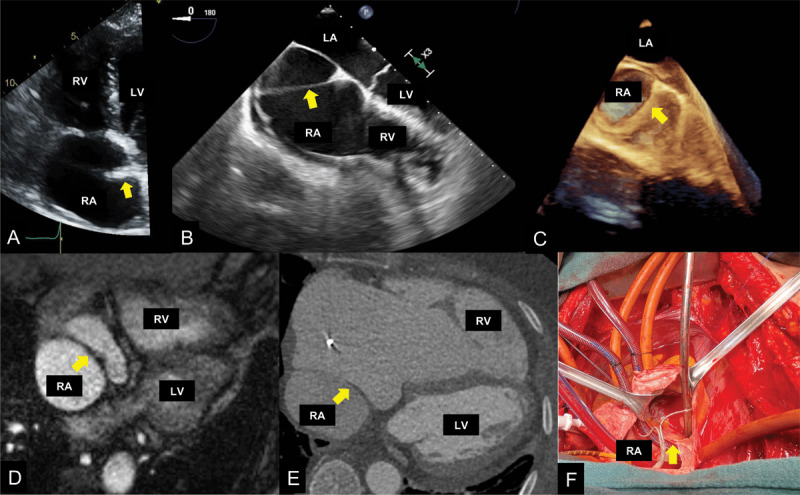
(A) Transesophageal echocardiogram (TEE) depicts a noncontinuous linear structure that seems to attach to the intra-atrial septum. (B) TEE further defines the membrane as a continuous structure, with color Doppler showing flow across membrane (see Video 1). (C) 3-dimensional (3D) TEE confirms what was seen in 2D imaging. (D) Cardiac magnetic resonance imaging confirms cor triatriatum dexter. (E) Coronary computed tomography angiography depicts low-attenuation structure traversing through right atrium. (F) Intraoperatively, cor triatriatum dexter was confirmed, but importantly, right ventricular inflow obstruction was excluded. RV: right ventricle; LV: left ventricle; LA: left atrium; RA: right atrium
